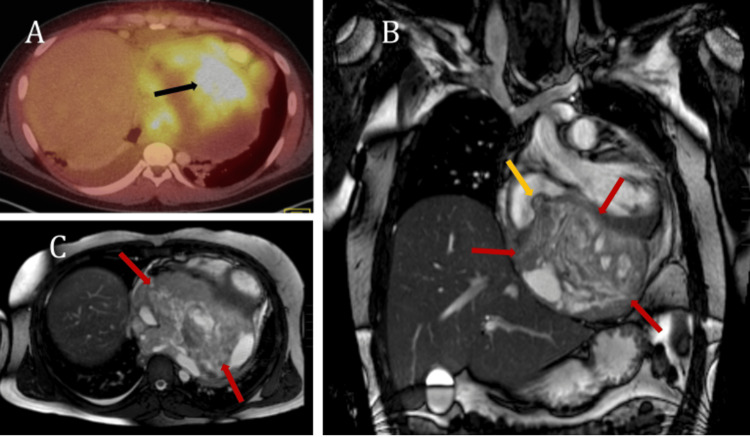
(A) 18F-FDG PET/CT scan with intense tracer uptake in this mass (black arrow in A). (B) Coronal T2W MRI of the chest without contrast with a large heterogeneous pericardial mass (red arrows in B), extending into the right atrium (yellow arrow in B). (C) Axial T2W MRI of the lower chest with heterogeneous pericardial mass (red arrows in C). Significant compromise of cardiac function was noted. 18F-FDG PET/CT: fluorine-18 fluorodeoxyglucose positron emission tomography/computed tomography, T2W: T2-weighted, MRI: magnetic resonance imaging
